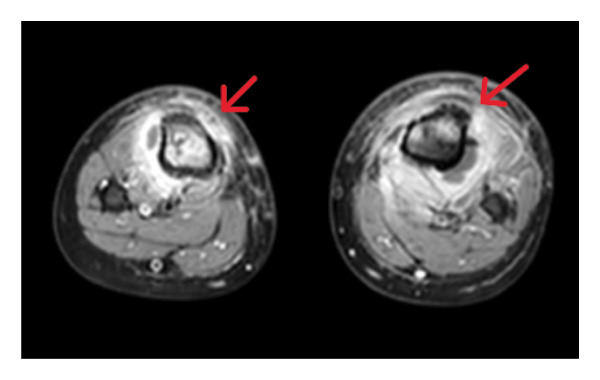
MRI of the lower limbs (T1‐weighted postcontrast) demonstrating multiple areas of low signal intensity with heterogeneous postcontrast enhancement involving the epiphysis and metaphysis of both proximal tibiae. The surrounding muscle compartments of both legs show heterogeneous edema with associated enhancement.
